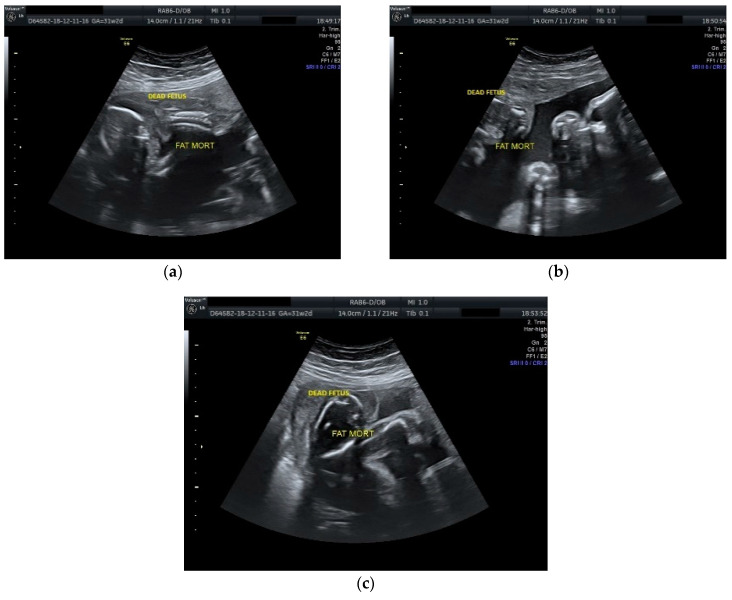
Ultrasound scan performed at 31 weeks and 2 days of gestation: (a), (b), (c) dead fetus with consistent plastic changes along with normal growing fetus with normal amniotic fluid.
